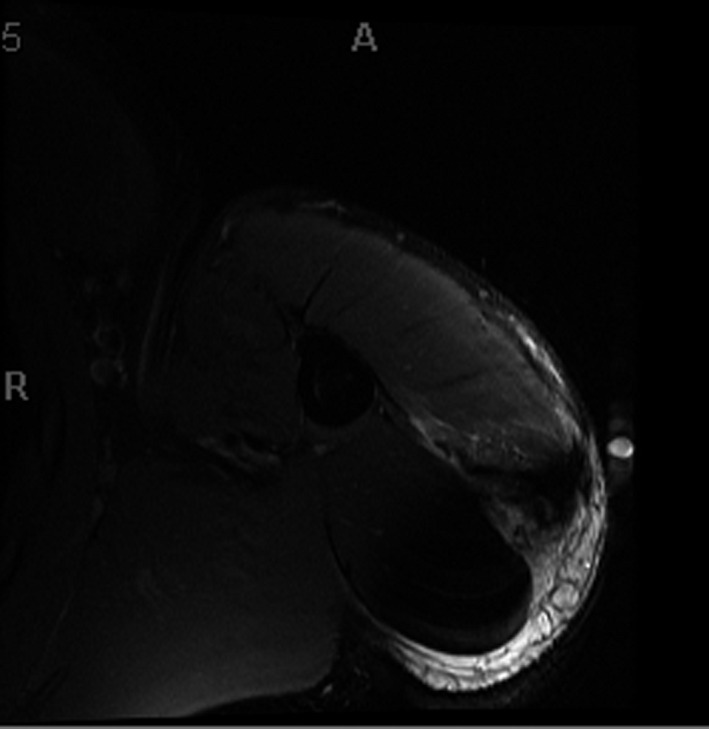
Left shoulder MRI axial STIR image demonstrating a posterior head of the deltoid muscle‐tendon avulsion from the deltoid tuberosity of the humerus. There is approximately 3 cm retraction of the torn fibers. There is associated muscle strain of the posterior head of the deltoid with hematoma formation
